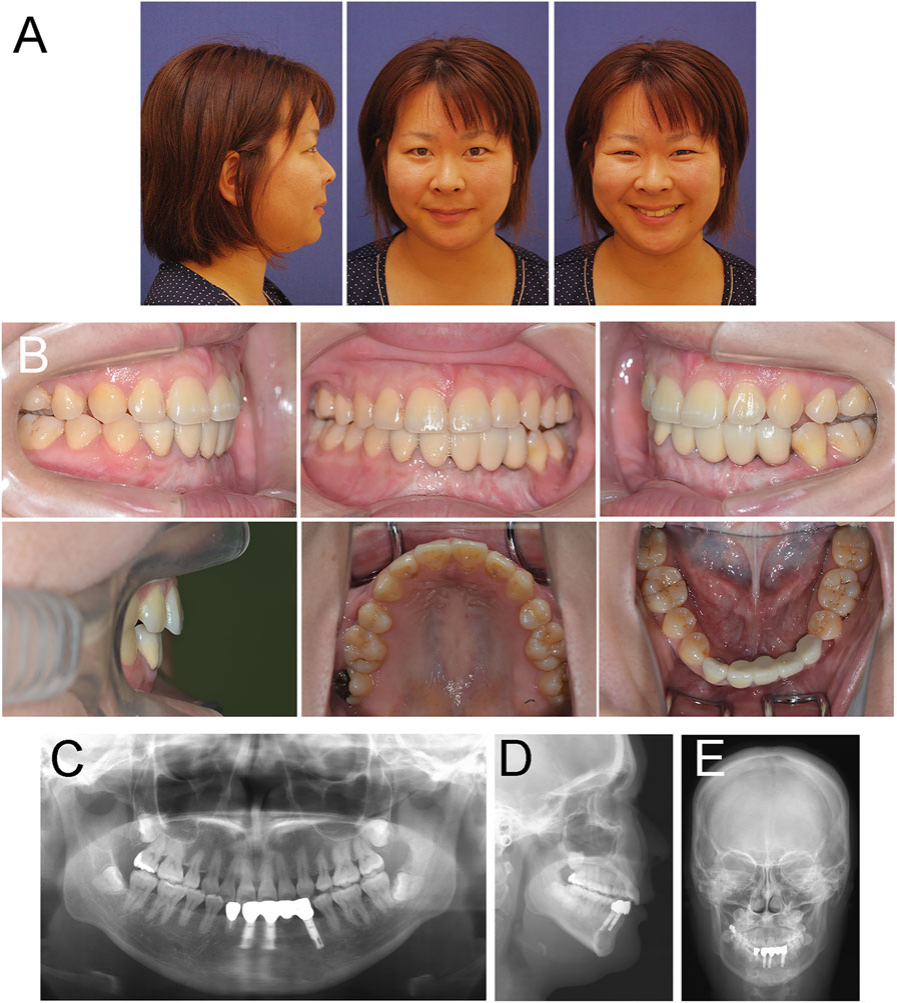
Two-year retention recordings. a Extraoral photographs. b Intraoral photographs. c A panoramic radiograph. d A lateral cephalogram. e A posteroanterior cephalogram
